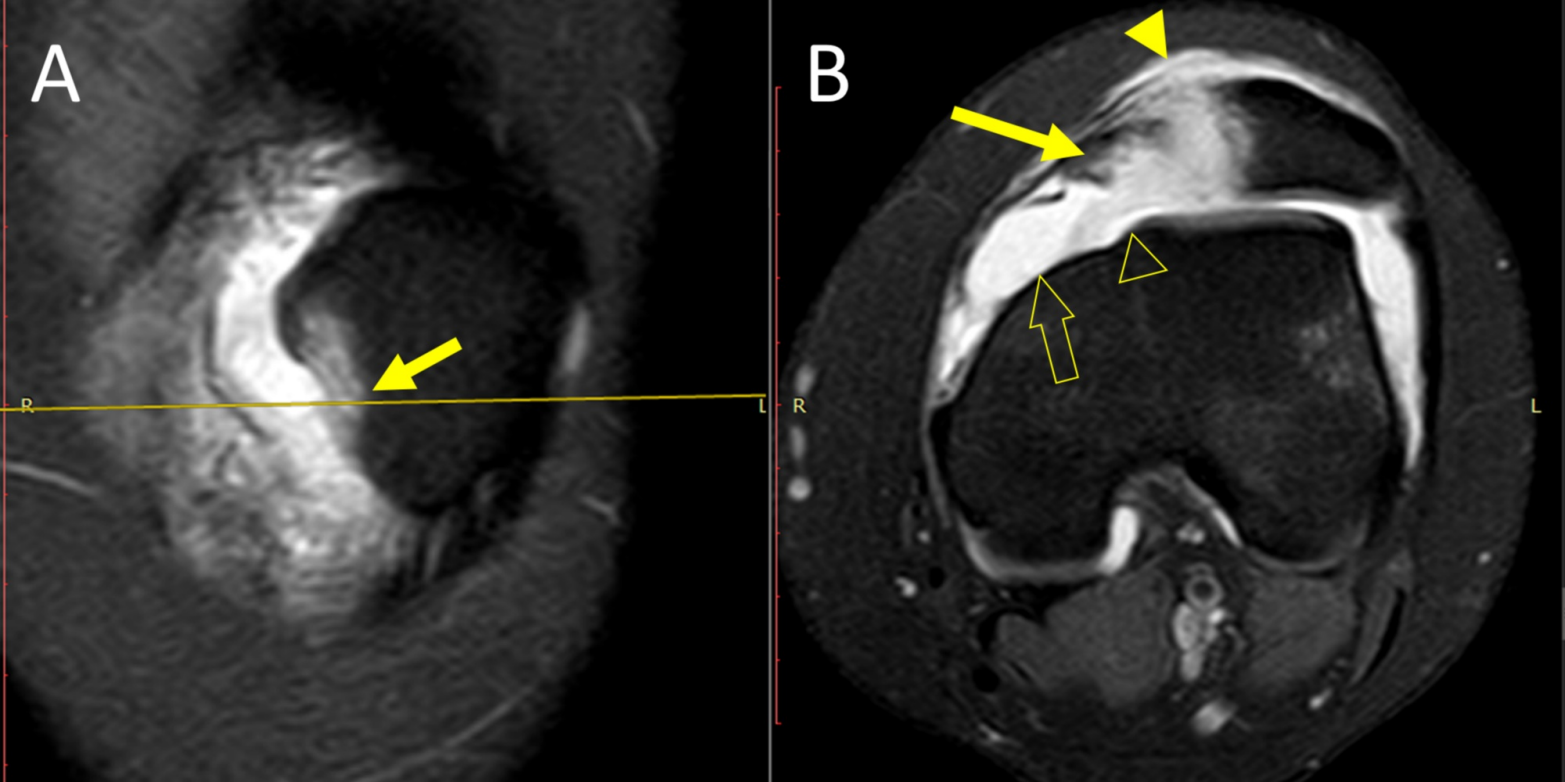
MRI of left knee: patellar attachment of MPTL and MPML A (frontal plane): the arrow indicates the location of avulsion fracture at the distal part of the medial patellar border. B (axial plane, 12 mm distal to the plane of figure 3B): patellar avulsion fracture (solid arrow), complete avulsion of distal medial restraints from the patella (solid arrowhead), hypoplastic medial femoral condyle (arrow outline), flat sulcus (arrowhead outline) MRI: magnetic resonance imaging; MPTL: medial patellotibial ligament; MPML: medial patellomeniscal ligament
